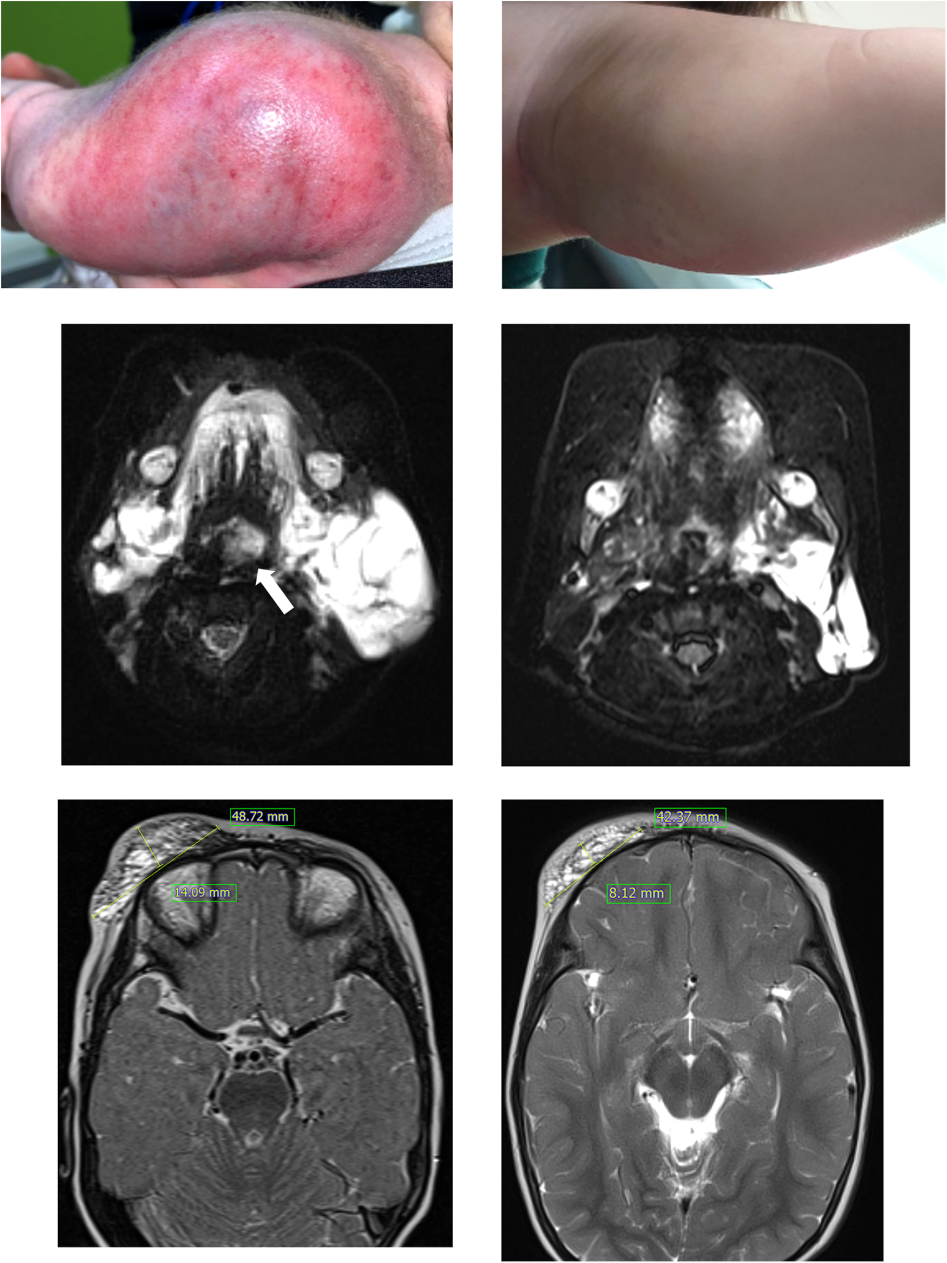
Patient 1 had a large kaposiform hemangioendothelioma on her left upper arm (A), and the mass showed improvement after nine months of sirolimus administration (B). In this T2-weighted MRI, patient 3, who presented with a large cystic mass on the lower left neck and around the airway (indicated by the arrow) at birth (C), experienced relief from airway compression after 5 months of sirolimus treatment (D). Patient 4 showed a protruding mass suspected to be a lymphatic malformation on the right forehead and eyelid (E), and a T2-weighted MRI was obtained after 6 months of sirolimus treatment (F).
